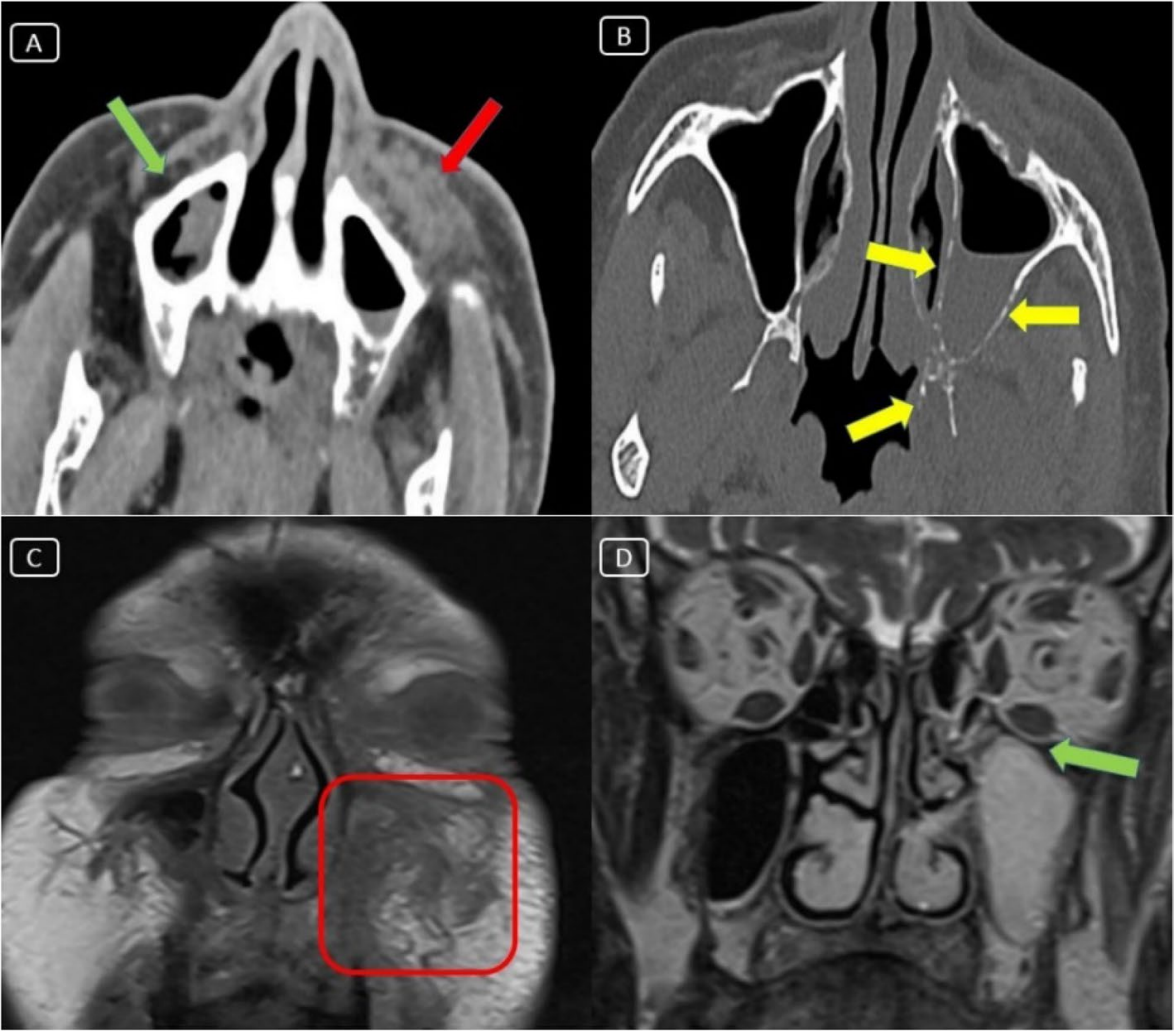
A, B Axial CT cuts of the paranasal sinuses and orbital regions revealed (1) soft tissue fullness replacing the left pre-maxillary antral fat (red arrow), (2) mild antral mucosal thickening, and (3) thinning out of the medial and lateral maxillary bony walls with areas of dehiscence and rarefaction that extended posteriorly up to the root of the pterygoid plates (yellow arrows). C Coronal T1WI MRI of the paranasal sinuses revealed asymmetrical fullness and heterogeneous signal intensity of the premaxillary fat (red square). D Coronal T2WI MRI of the paranasal sinuses and orbital regions revealed left maxillary sinusitis still with clear peri-orbital fat planes (green arrow)
